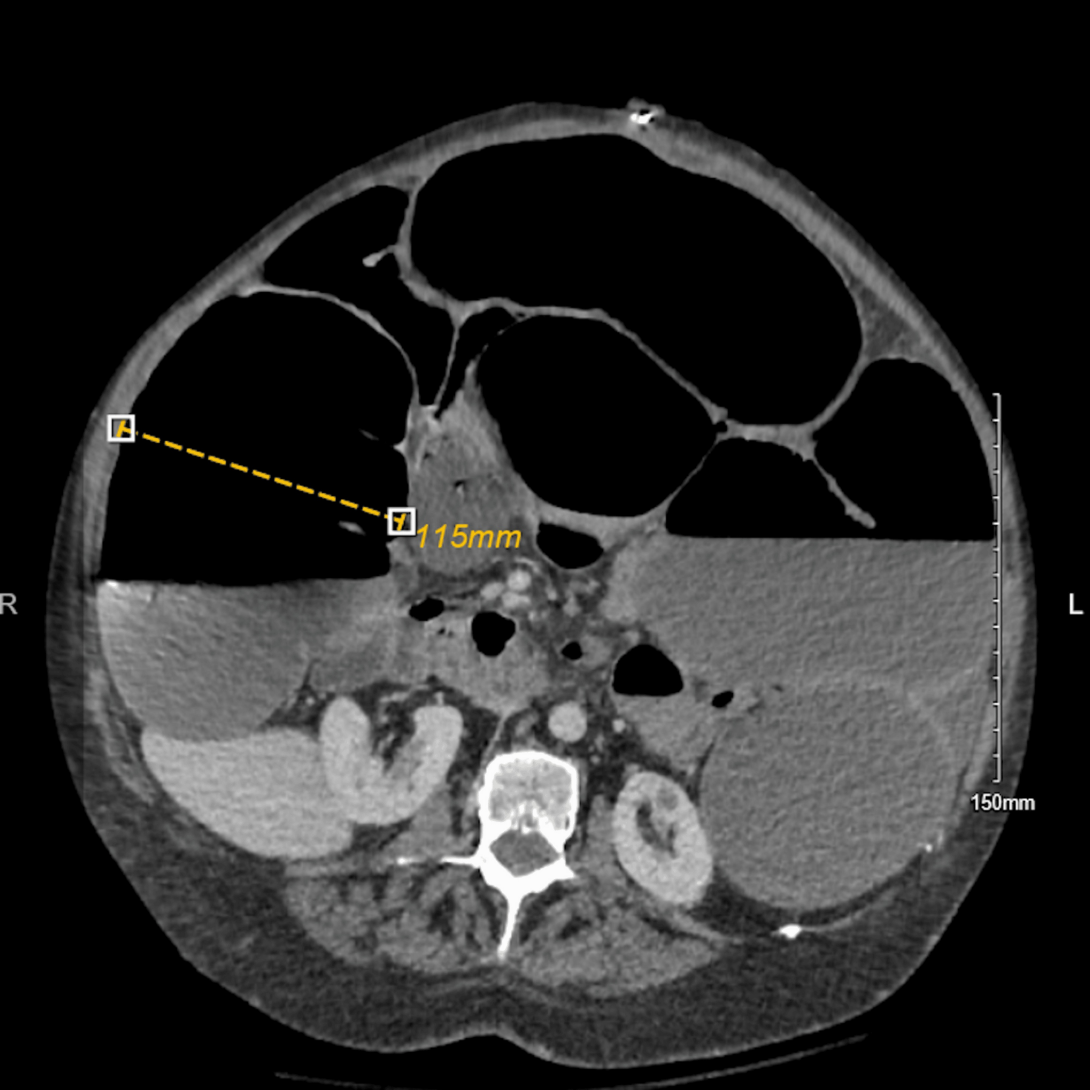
Axial CT of the abdomen demonstrating a markedly distended large bowel loop measuring 11.5 cm, as indicated by the dotted yellow line.
